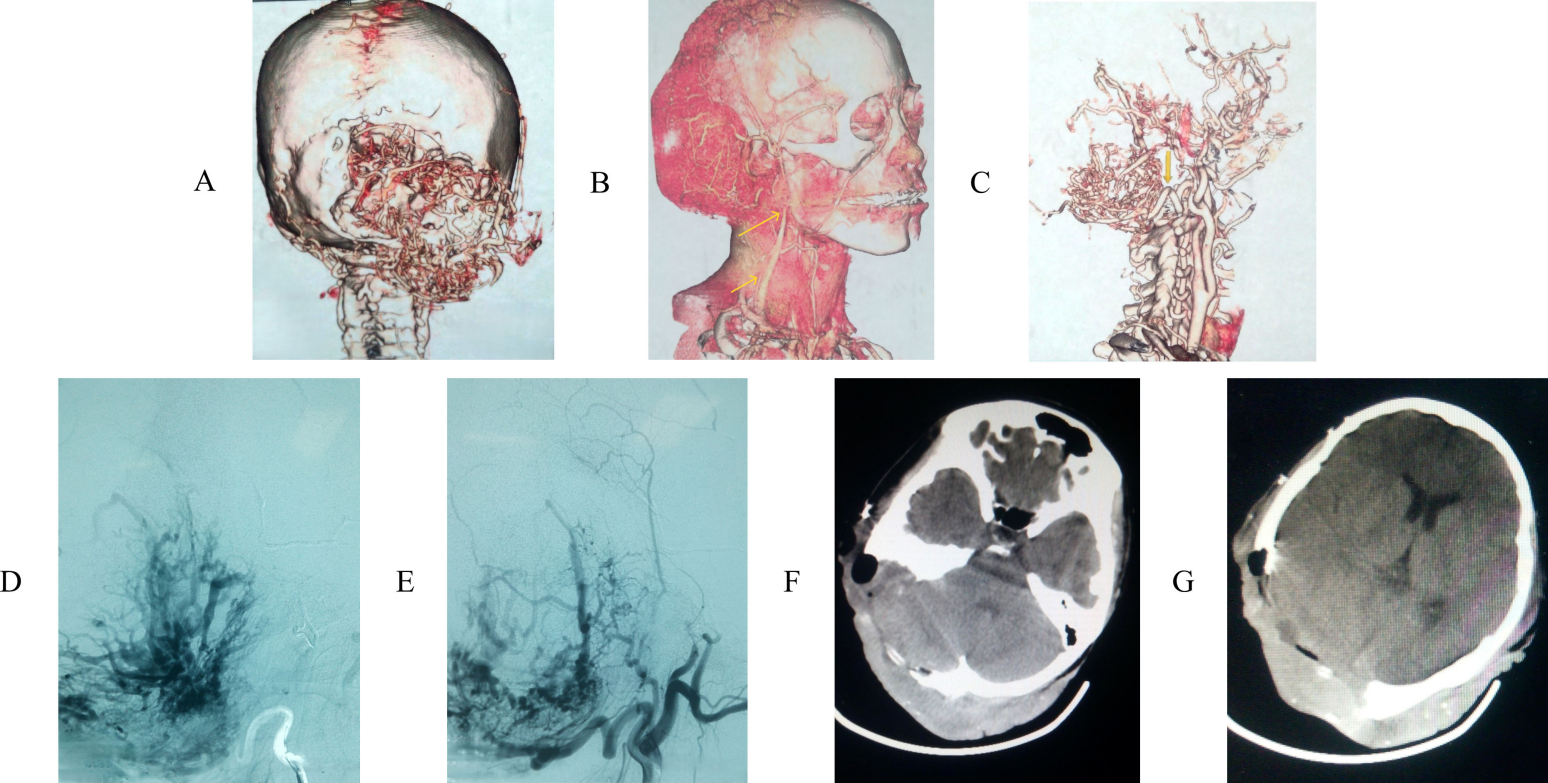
(A) CT angiography (CTA) with 3D reconstruction revealed tumor was hypervascula, leading to an angiomatous lesion with skull invasion. (B) CTA presented the lesion’s vascularization. (C) the lesion’s main vascularization were occipital artery (bottom arrow) and arteriae auricularis posterior (upper arrow). (D, E) the patient accepted embolization therapy of occipital. (F, G) most parts of the tumor were removed.
